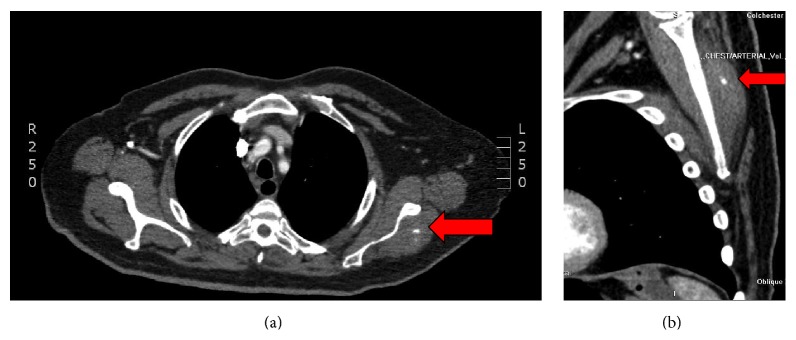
Axial and oblique CT scan, respectively, demonstrating lesion within the left infraspinatus muscle (arrow). Area of central calcification evident.
